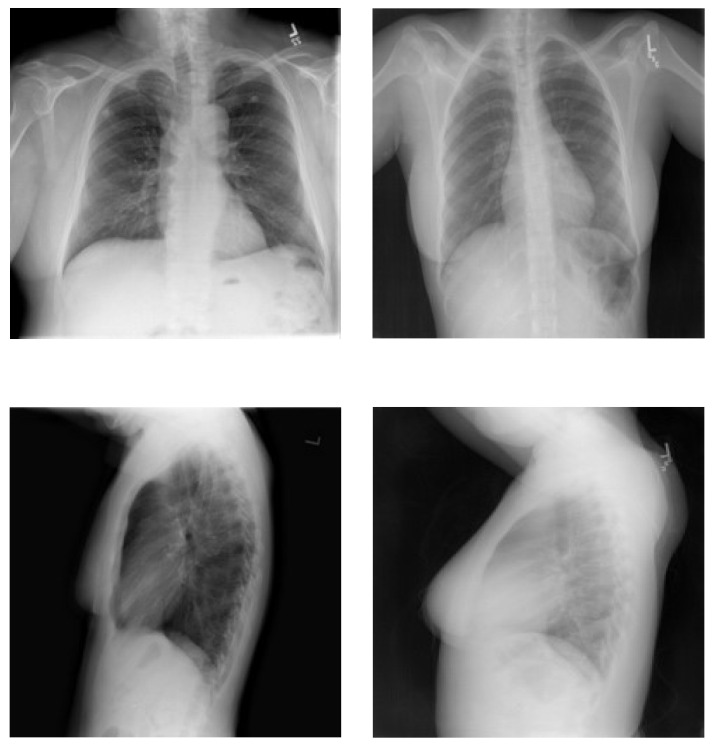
Turkish chest X-ray images reporting dataset randomly selected sample images.
